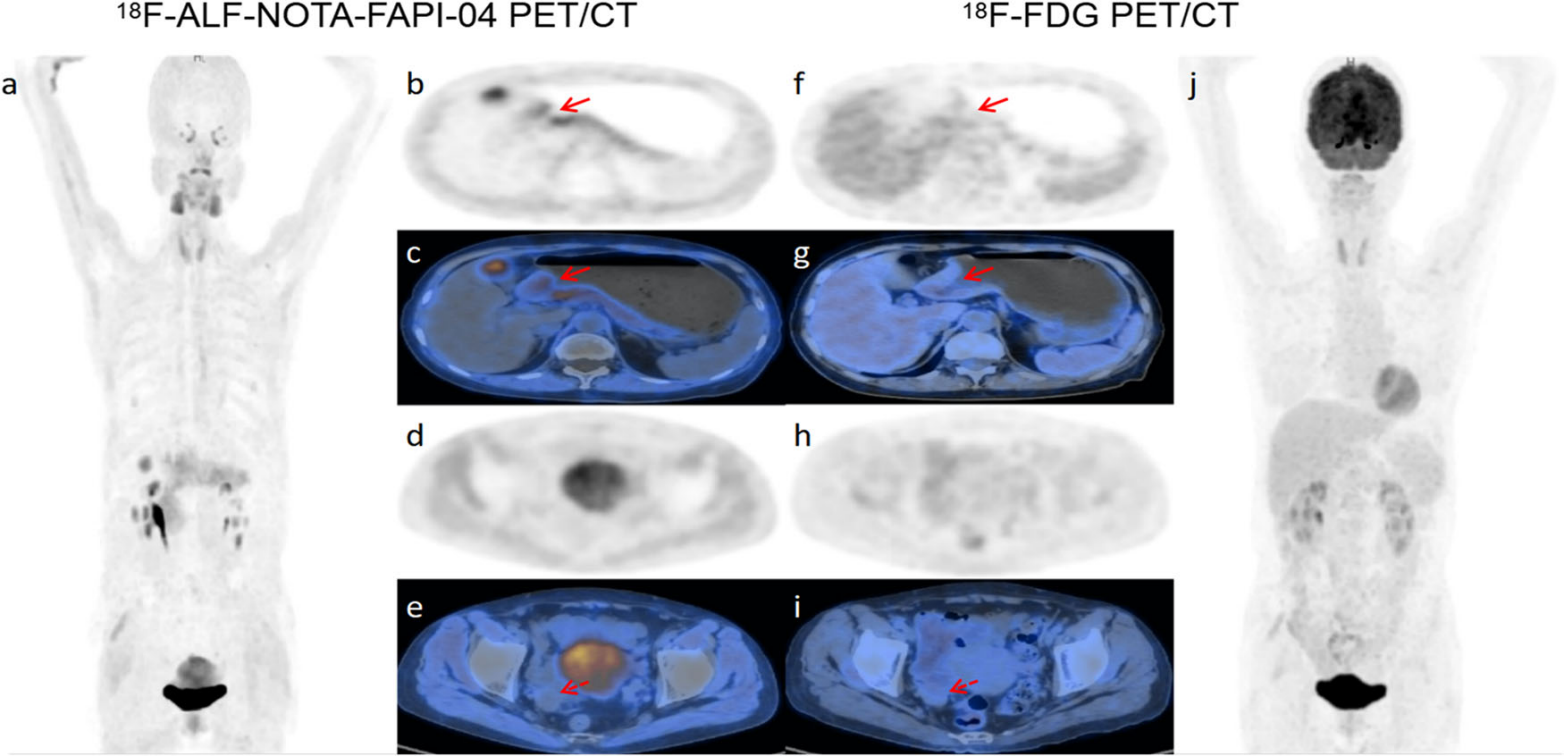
A 56-year-old female with poorly differentiated adenocarcinoma underwent follow-up examination after 10 cycles of chemotherapy. [^18^F]ALF-NOTA-FAPI-04 PET/CT (a–c) demonstrated moderate uptake in the gastric antrum and angulus (solid arrows, SUVmax = 3.9), while [^18^F]FDG PET/CT (f, g) showed mild uptake (solid arrows, SUVmax = 2.5). Postoperative pathology confirmed residual diffuse and multifocal moderate-poorly differentiated adenocarcinoma tissue. Surgical pathology of the right ovary also confirmed the presence of poorly differentiated adenocarcinoma, consistent with the Krukenberg tumor. Both [^18^F]ALF-NOTA-FAPI-04 PET/CT (d, e) and [^18^F]FDG PET/CT (h, i) revealed negative uptake (dotted arrows)
